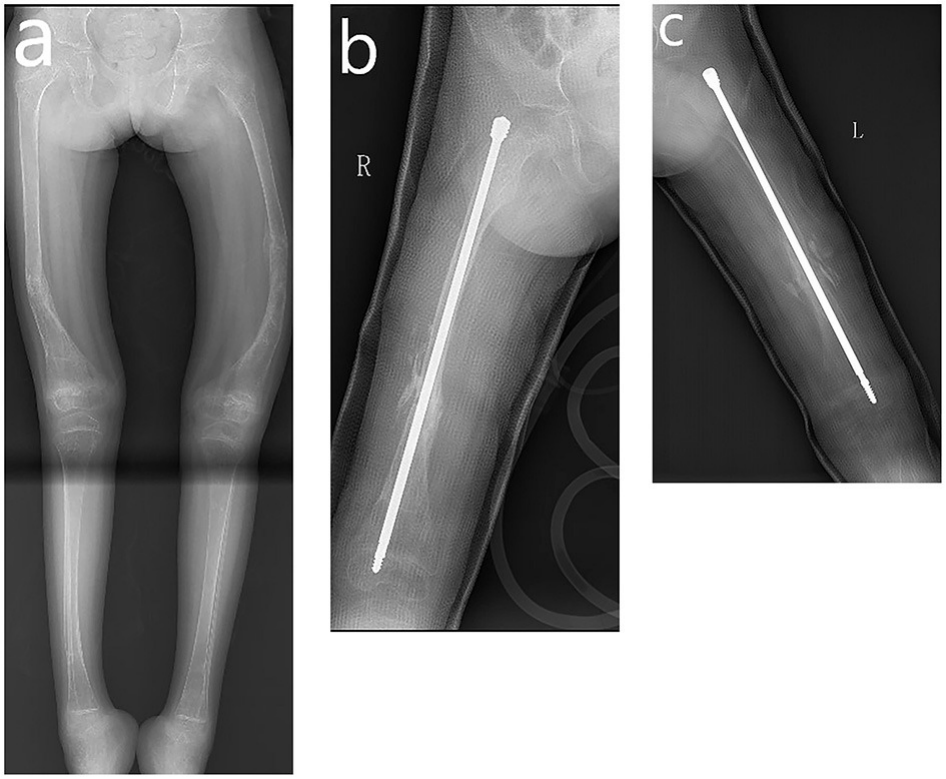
Radiograph of fractures in lower extremity. (a) Full-length image of the lower extremities showing fracture and bowing in bilateral femur. (b) Nailing of the right femur. (c) Nailing of the left femur.
